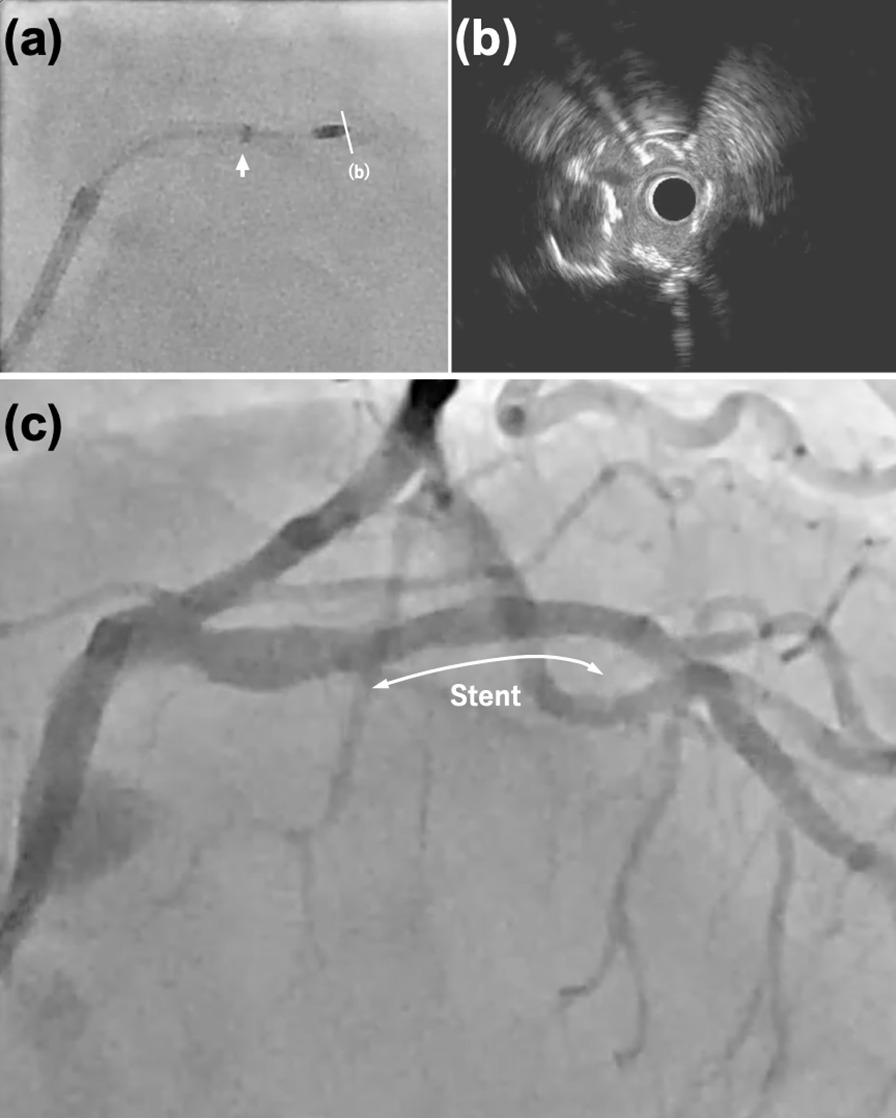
Coronary angiography and intravascular ultrasound (IVUS) imaging. a Rotational atherectomy supported by the Guidezilla II (arrow). b IVUS view (a, white line) showing a cracked calcified nodule at 7 o’clock. c Final result after stent (bidirectional arrows) deployment
